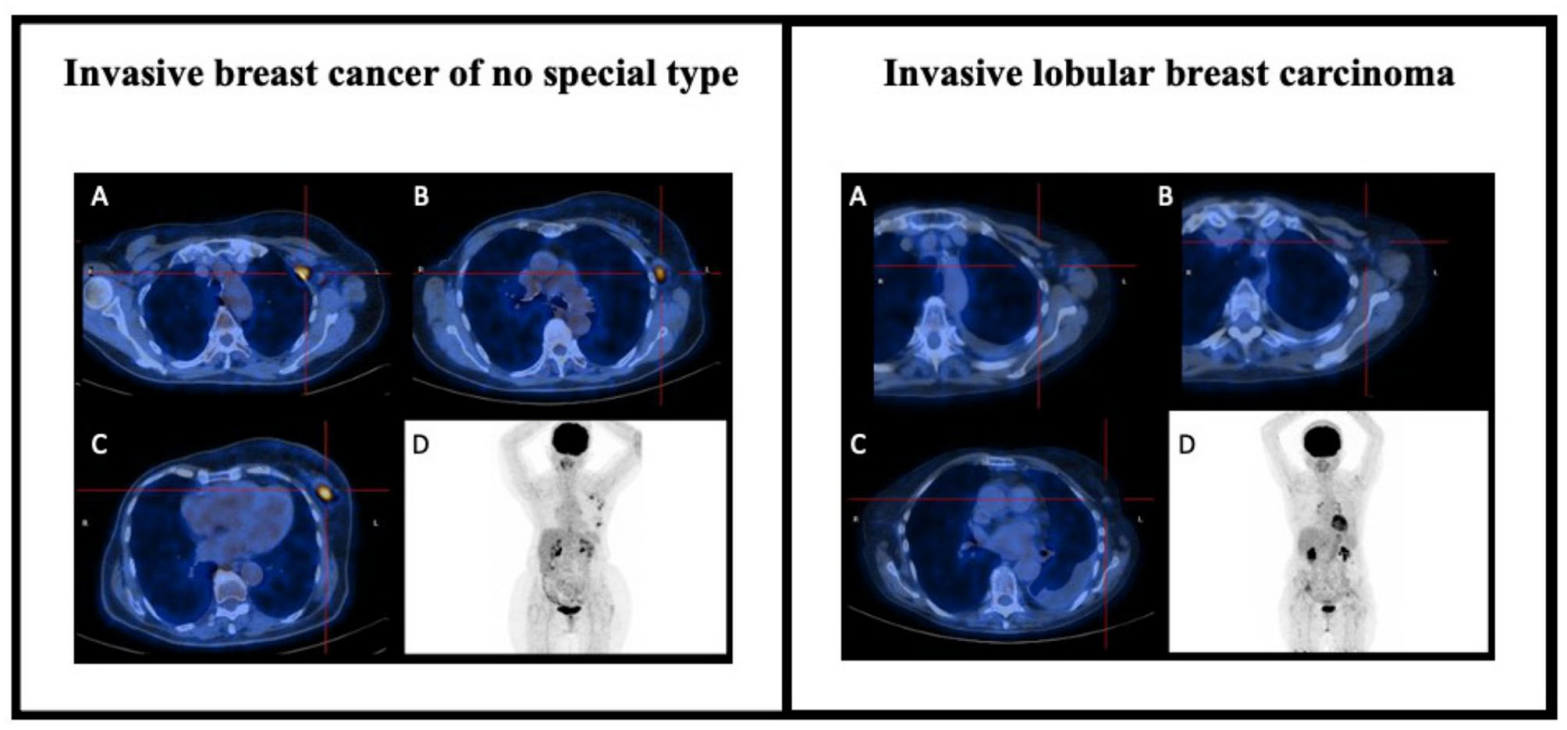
[^18^F]F-FDG PET/CT in BC staging. On the left, the case of an 83-year-old lady with previous *in situ* BC on the right and suspected recurrence of breast cancer on the left. Staging [^18^F]F-FDG PET/CT scan showed focal and elevated uptake at the level of the breast lesion on the left upper-external quadrant (C) and at the level of several ipsilateral axillary lymph nodes suspected for secondary localization of disease (A,B), as also shown in the MIP images (D). The cytologic finding was consistent with the clinical suspicion of IBC-NST recurrence (ER 98%, PR 99%, anti-Ki67 23%, anti c-erbB2 negative). On the right, the case of a 72-year-old lady hospitalized for dyspnea and CT findings of right pleural effusion concomitant to left mammary nodule and mediastinal lymphadenopathies. Staging PET scans did not show any FDG uptake in the suspicious lesions reported on CT scan, either in the breast (C) or in the ipsilateral axillary lymph nodes (A,B), as also shown in MIP images (D). The cytological findings were consistent with the clinical suspicion of ILBC (ER 78%, PR 55%, anti-Ki67 46%, anti c-erbB2 negative).
